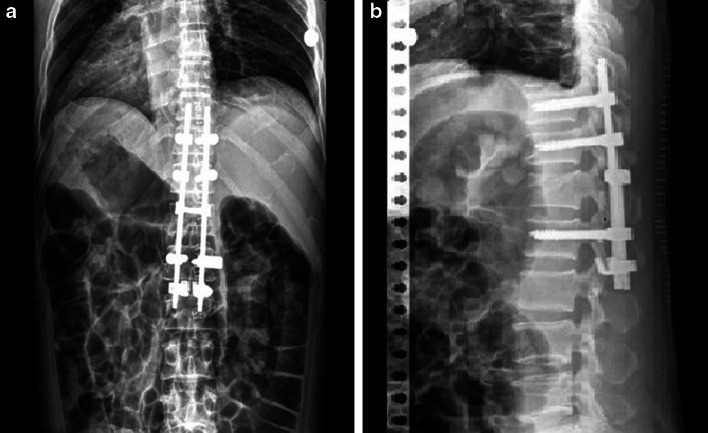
a Postero-anterior and b lateral radiographs of a patient with an L1 vertebra fracture who received IS instrumentation
